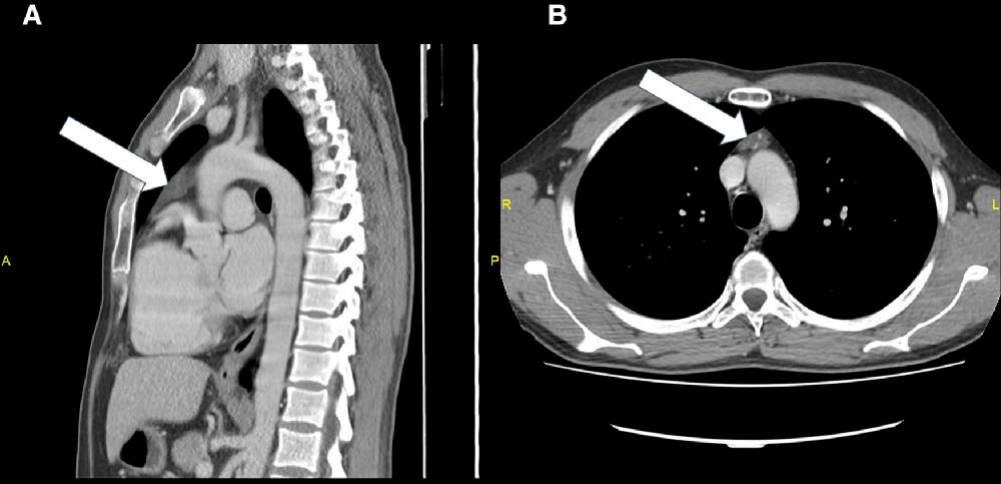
A and B, Computed tomography image at the time of diagnosis showing the enlarged thymus. A, Lateral and B, axial plane views.
